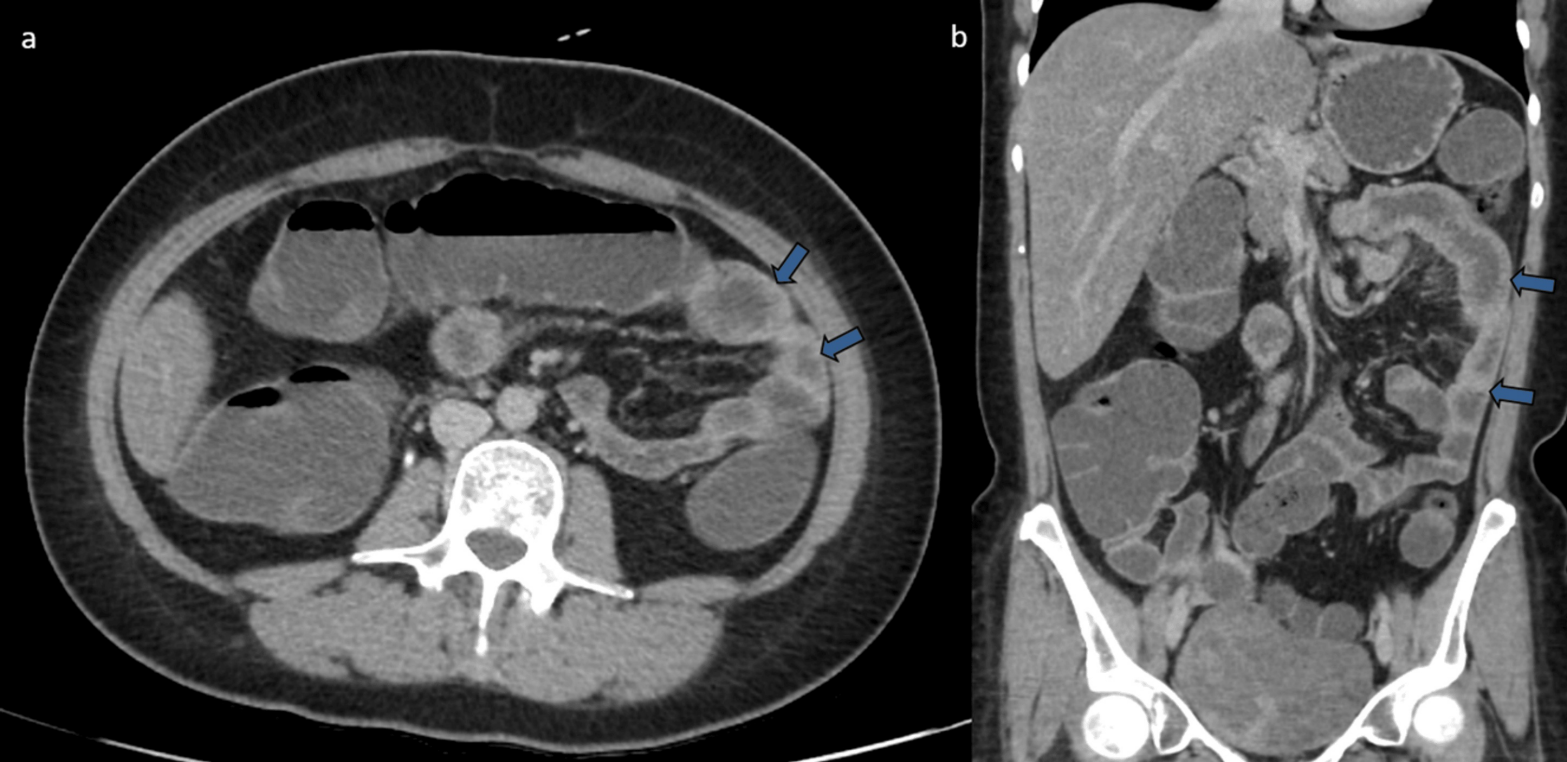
Computed tomography (CT) enterography performed seven days after angiotensin-converting enzyme inhibitor suspension. In the axial (a) and coronal section (b), we can see normal distensibility of jejunal and ileal loops, without dilated or thickened small bowel segments (arrows). No peritoneal effusion.
